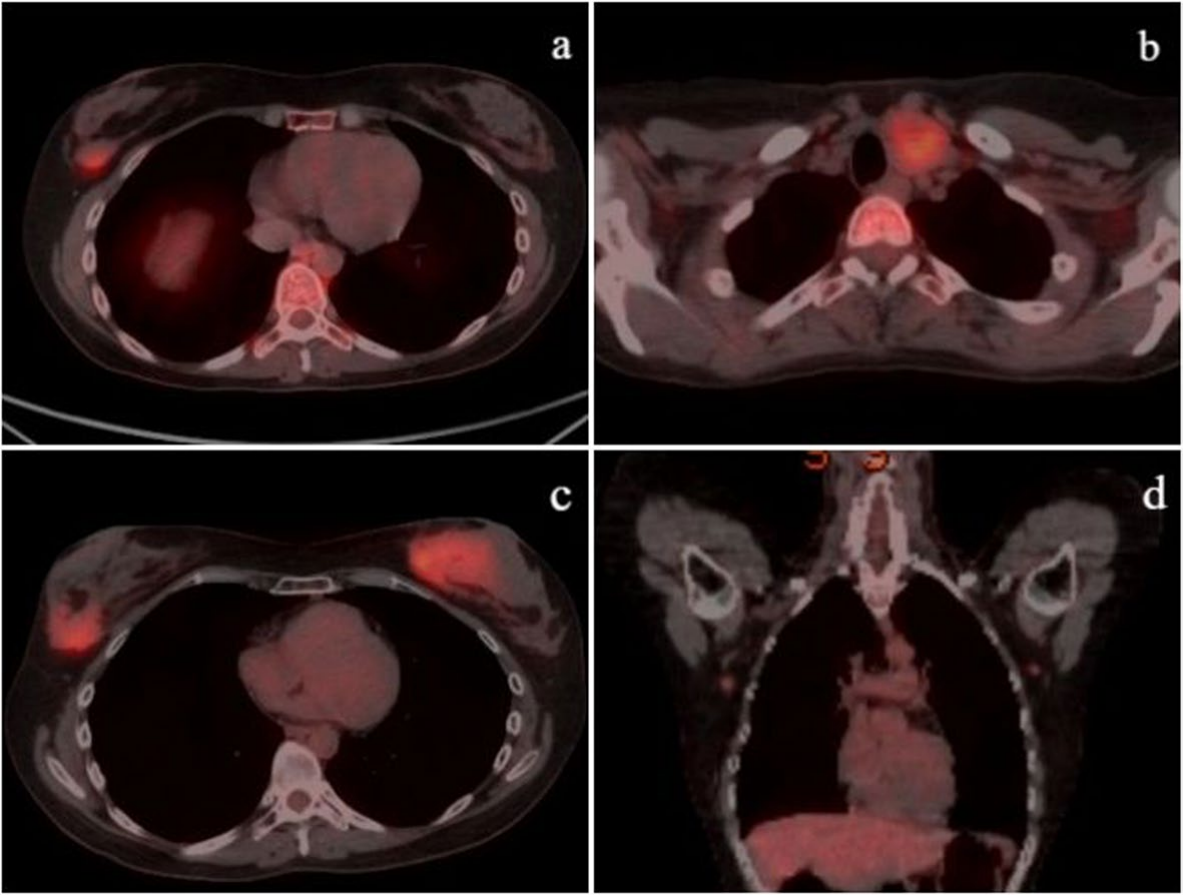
FDG-PET images of breast and thyroid lesions. a Lesion showing increased FDG uptake in the right mammary gland at diagnosis (size 2 cm, SUV max 3.9). b Lesion showing increased FDG uptake (SUV max 5.7) in the left lobe of the thyroid gland at diagnosis. c Lesion in the right breast showing increased size and metabolism compared to previous FDG-PET (size 4.2 cm, SUV max 9.6) and a newly developed lesion in the left breast. d Lymphadenopathies showing increased FDG uptake in both axillae (size 1 cm, SUV max 3.7)
